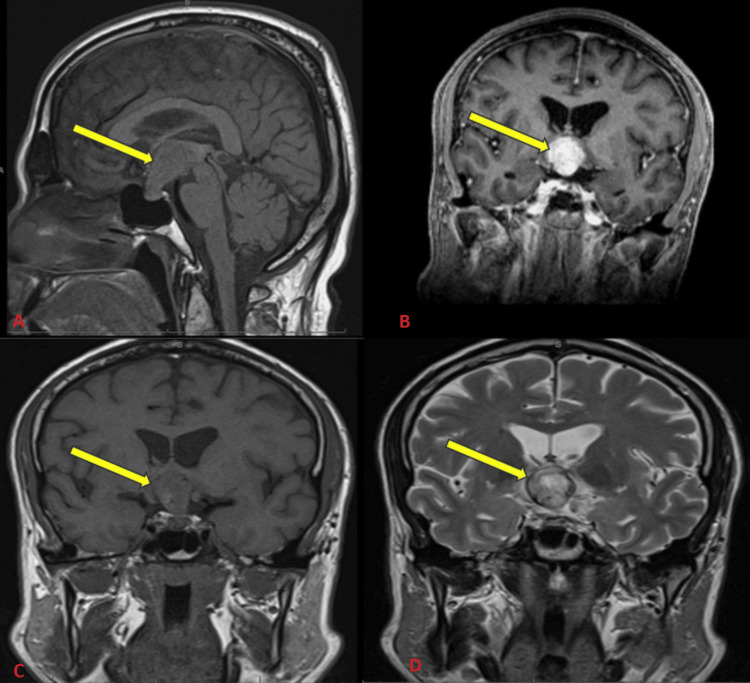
MRI of the brain showing sellar and suprasellar mass measuring 2.1 × 2.3 × 3.8 cm, extending to the suprasellar cistern, and elevating the optic chiasm. A: T1-weighted sagittal view showing hypointense pituitary macroadenoma (yellow arrow). B: T1-weighted coronal view post intravenous contrast diffuse enhancement in the sella region (yellow arrow). C: T1-weighted coronal view showing hypointense macroadenoma (yellow arrow). D: T2-weighted coronal view showing a heterogeneous signal with a rim of hemosiderin and internal heterogeneous iso and hyperintense areas (yellow arrow).
